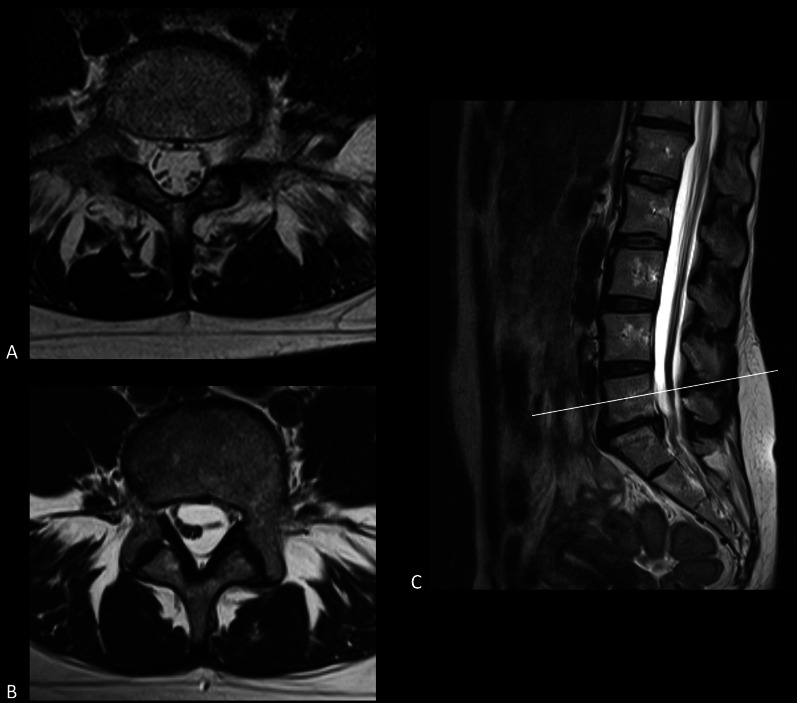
T2-weighted MRI of the same FBBS patient at two-time intervals. A First available axial T2-weighted MRI section at the level of the fifth lumbar vertebra. Cauda equina roots are seen to float in ample cerebrospinal fluid. B Axial T2-weighted MRI section at the level of the fifth lumbar vertebra of the same patient after spinal intervention at level L5–S1. The patient complains of recurrent right-sided leg pain, sensory changes, and motor weakness. MRI show new clumping of the cauda equina roots on the right side of the dural sac. These features were consistent with arachnoiditis. C Mid-sagittal T2-weighted MRI, which also shows the clumping of cauda equina roots
